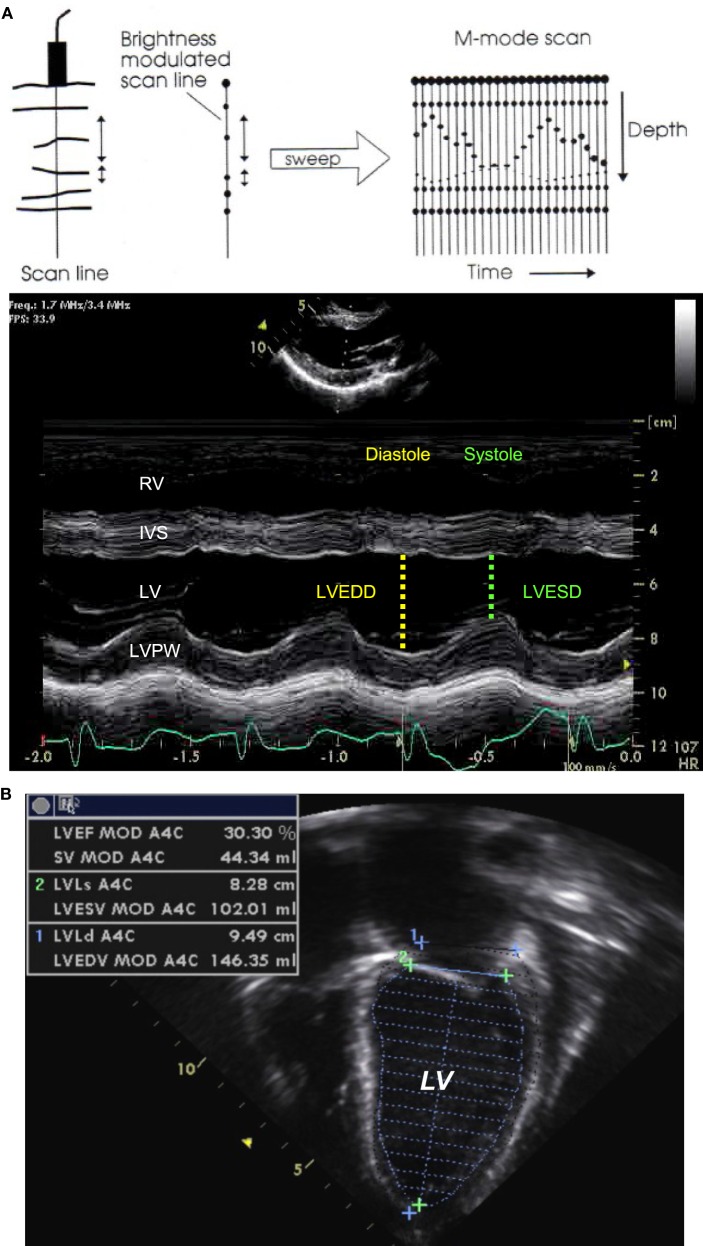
M-mode echocardiography (A) obtained from the parasternal long axis view through the right and left ventricular chambers at the tip of the mitral valve leaflets, allowing for the estimation of the fractional shortening. Two-dimensional echocardiography (B) obtained from an apical four-chamber view with tracing of the endocardial LV border during end-diastole and end-systole, allowing for estimation of the ejection fraction. IVS, interventricular septum; LV, left ventricle; LVEDD, left ventricular end-diastolic dimension; LVESD, left ventricular end-systolic dimension; LVPW, left ventricle posterior wall; RV, right ventricle. Adapted from Ref. (12).
